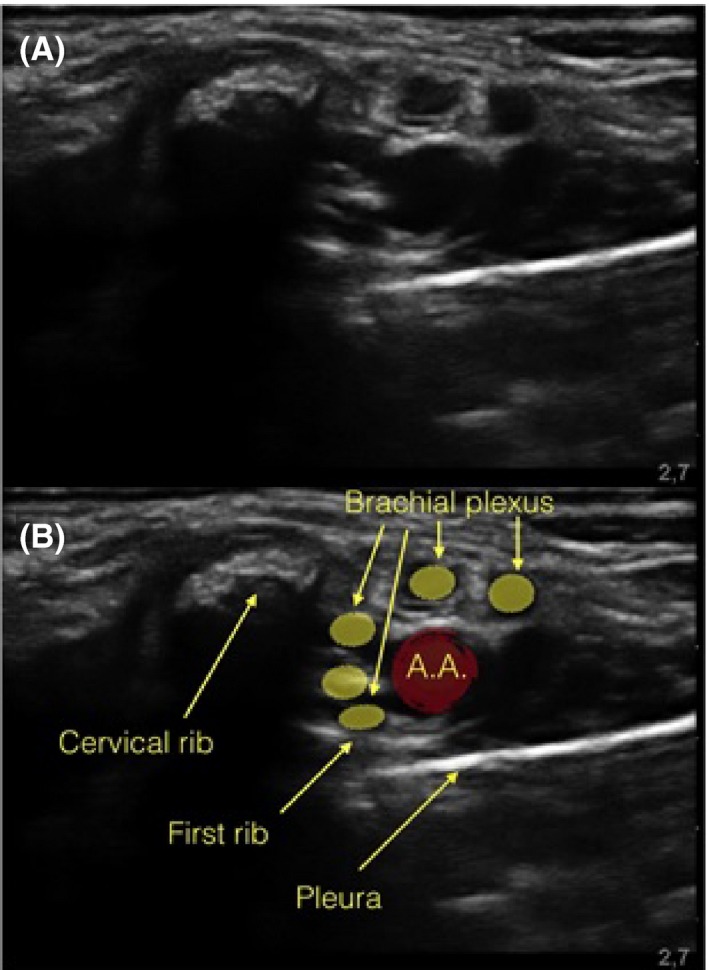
Supraclavicular approach of brachial plexus. A, The ultrasound image of the supraclavicular space. B, The same picture with labels of the anatomical structures AA, subclavian artery
